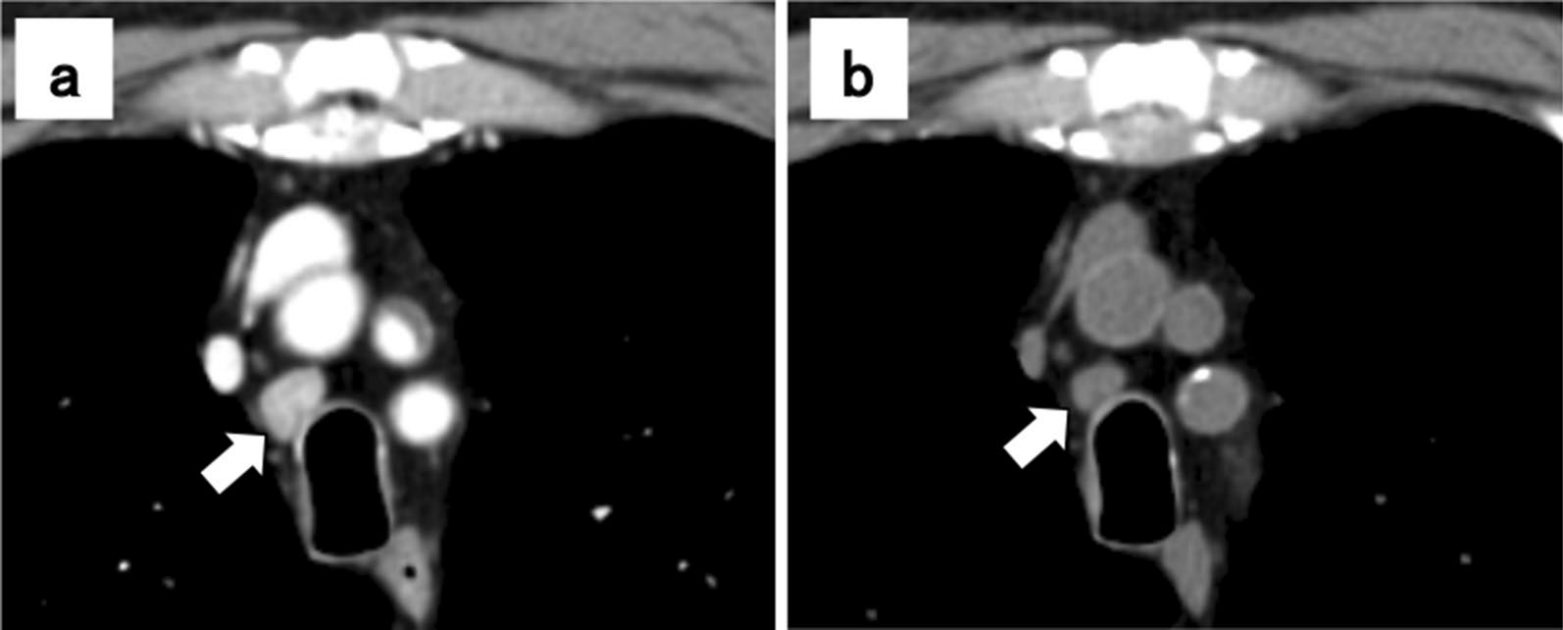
Computed tomography images of the upper mediastinal lymph node metastasis before (a) and after 2 months of lenvatinib treatment (b)
